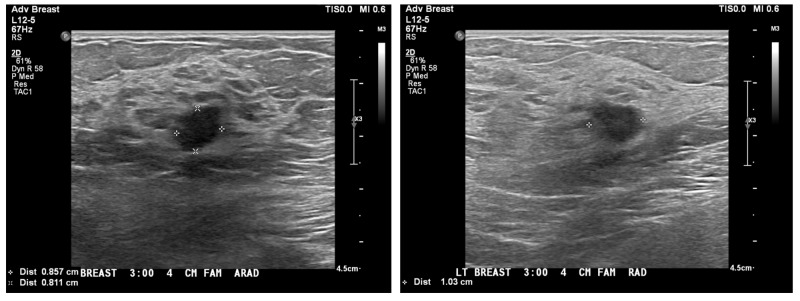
Ultrasound images showing the size of the the mass in the left breast
